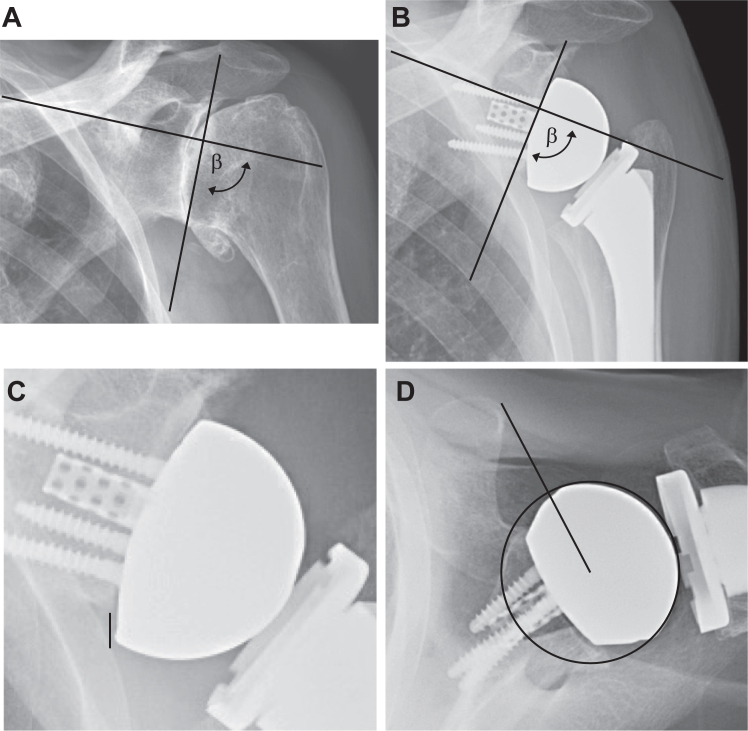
The measurement methods for (A) pre-operative inclination (β-angle), (B) post-operative β-angle, (C) inferior offset, and (D) center of rotation to the tip of the coracoid are demonstrated. Inclination, β-angle, and inferior offset were measured in true anteroposterior (Grashey) views. Center of rotation to the tip of the coracoid was measured with axillary views.2.Inferior offset (glenoid component overhang) as the vertical distance between the inferior margin of the native glenoid and the most inferior point of the glenosphere (Fig. 2*C*).^5^3.Center of rotation to the tip of the coracoid was the distance (mm) from the center of rotation to the posterior aspect of the coracoid on axillary radiographs as previously described (Fig. 2*D*).^14^
